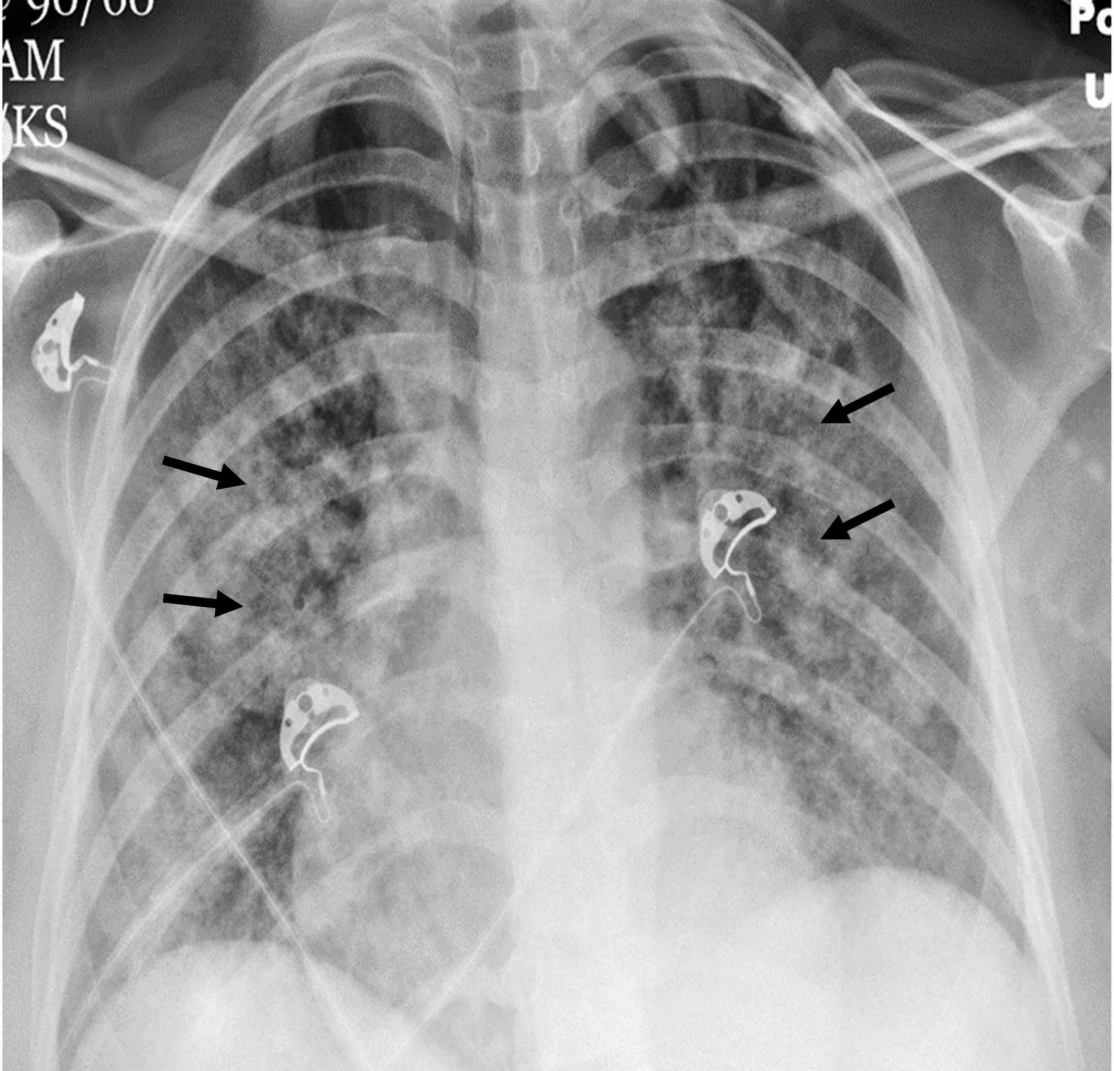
Chest X-ray Chest X-ray (AP view) showing bilateral alveolar opacities suggestive of airspace disease (black arrows)
